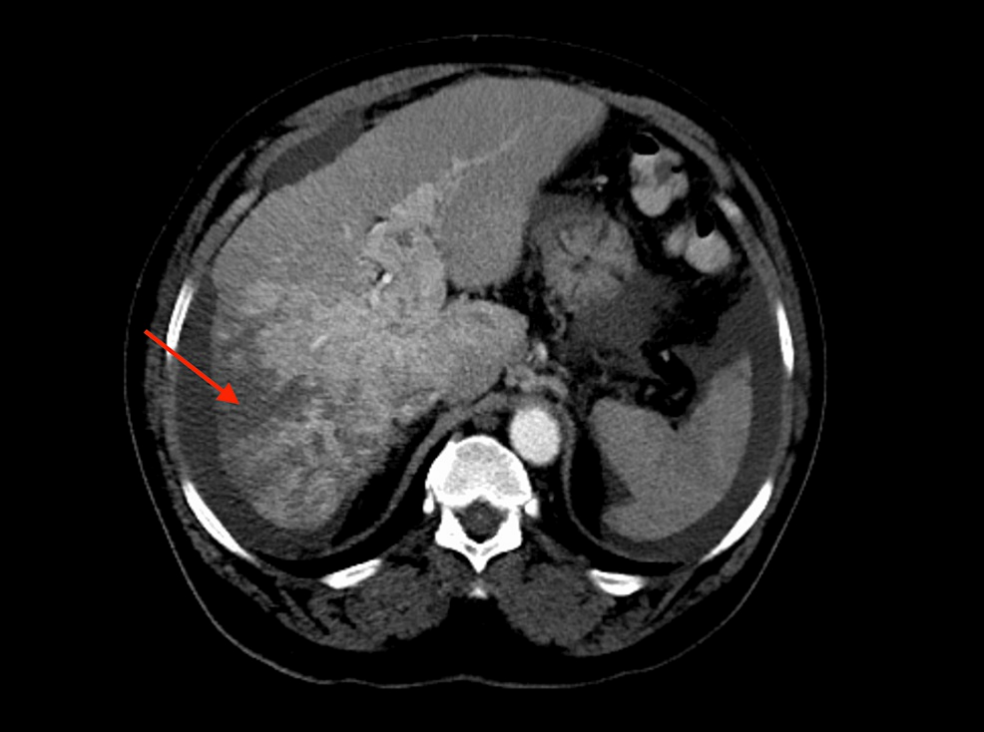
Transverse plane computed tomography (CT) image of the abdomen showing heterogenous liver lesion
